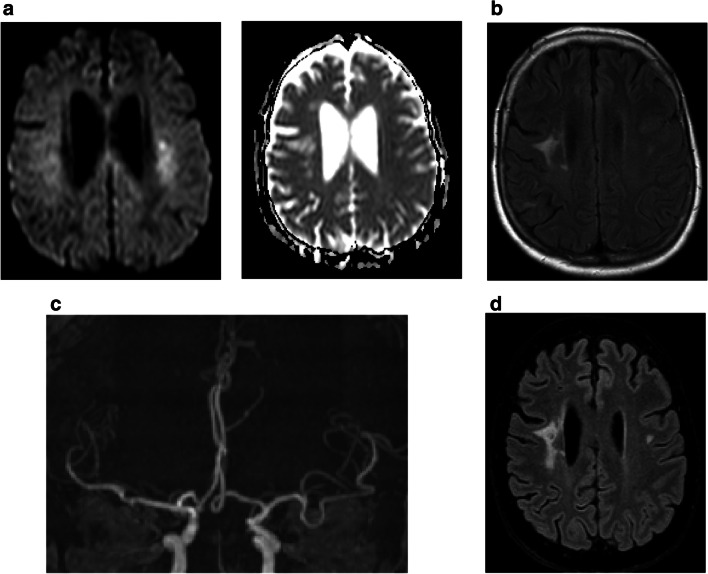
Patient was admitted with suspected cerebral vasculitis. MRI showed small restriction in diffusion within the left corona radiata (a, DWI left and ADC map right panel) as well as elder cortical and subcortical postischaemic lesions within the right hemisphere (b). TOF angiography revealed small arteria basilaris and bilateral occluded proximal arteria cerebri posterior (c), which were peripherally collateralized from anterior circulation. Since further diagnostic procedures were not fully conclusive, patient was planned for biopsy. Empiric treatment for vasculitis was initiated after non-diagnostic biopsy. Patient showed a clinical treatment response under empiric therapy and no new lesions in MRI at follow-up (d)
